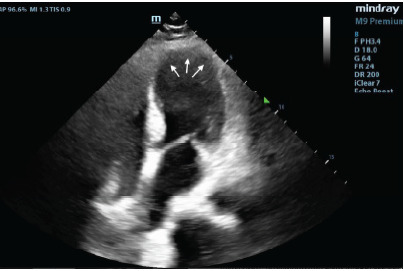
Echocardiogram of the apical four-chamber view using a low-frequency phased array probe showing left ventricular apical thinning with ballooning of the wall (arrows).
